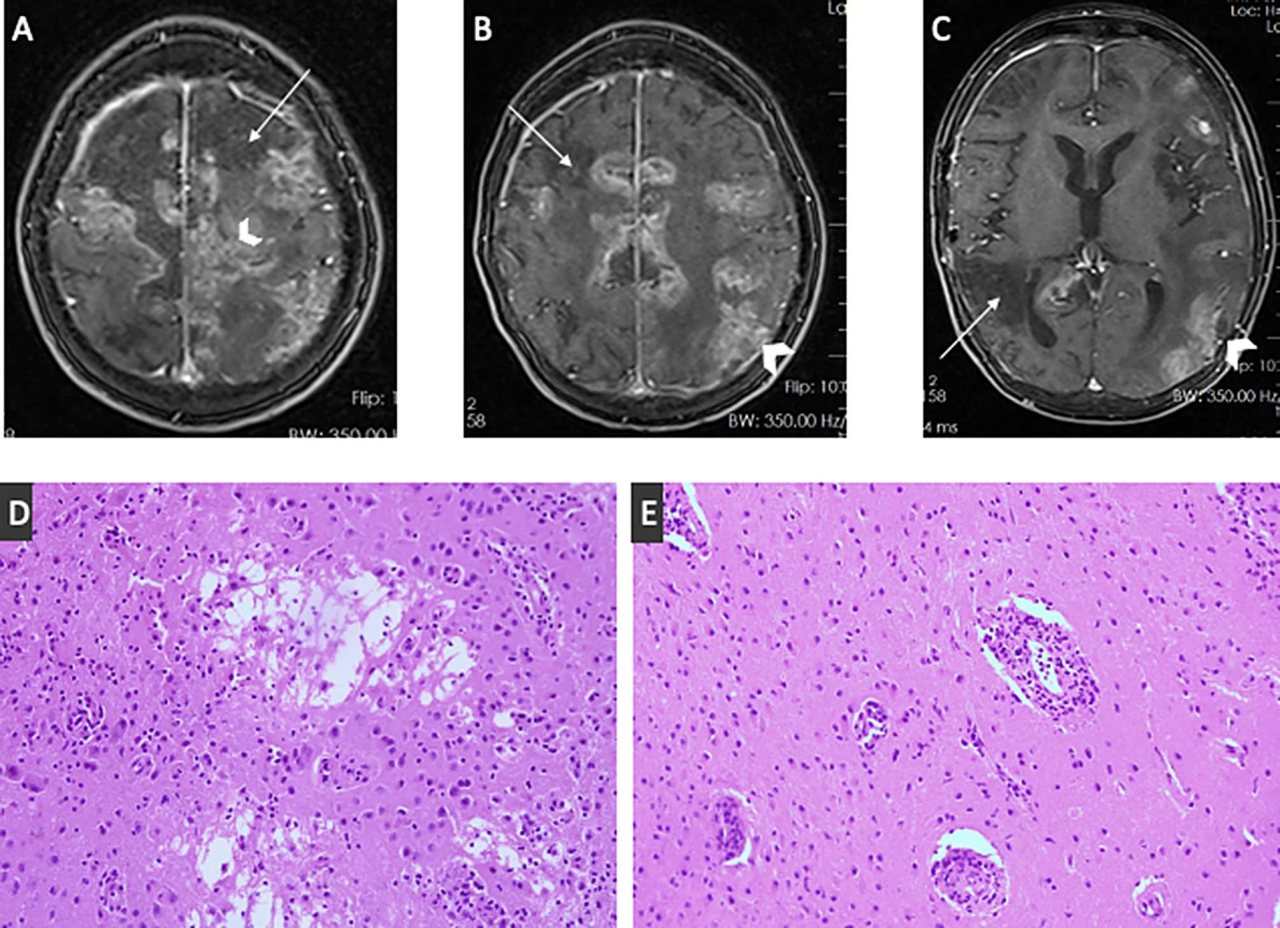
(A-C) contrast-enhanced axial T1-weighted fat-saturated MR images showed multiple wedge-shaped cortical-subcortical hypo-intensities in both hemispheres due to ischemic infarct (thin arrows) and patchy leptomeningeal and cortical enhancements (arrowheads). (D) microcystic formation and partial cavitation of brain parenchyma along with foci of chronic inflammation composed of lymphocytes and some macrophages in favor of encephalitis (HE x400). (E) transmural inflammation of the vessels with more than two layers of inflammatory cells mainly composed of lymphocytes in favor of vasculitis (HE x200)
